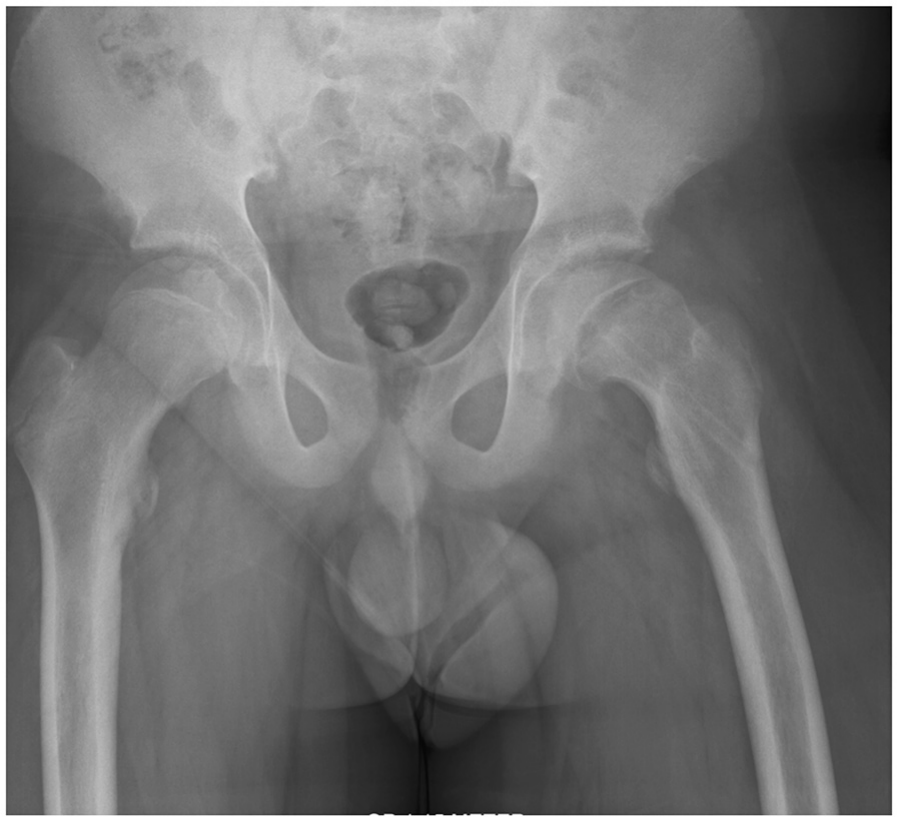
Patient 1. The anteroposterior X-ray after removal of the screw fixation shows progression of the slip to nearly 70 degrees.
